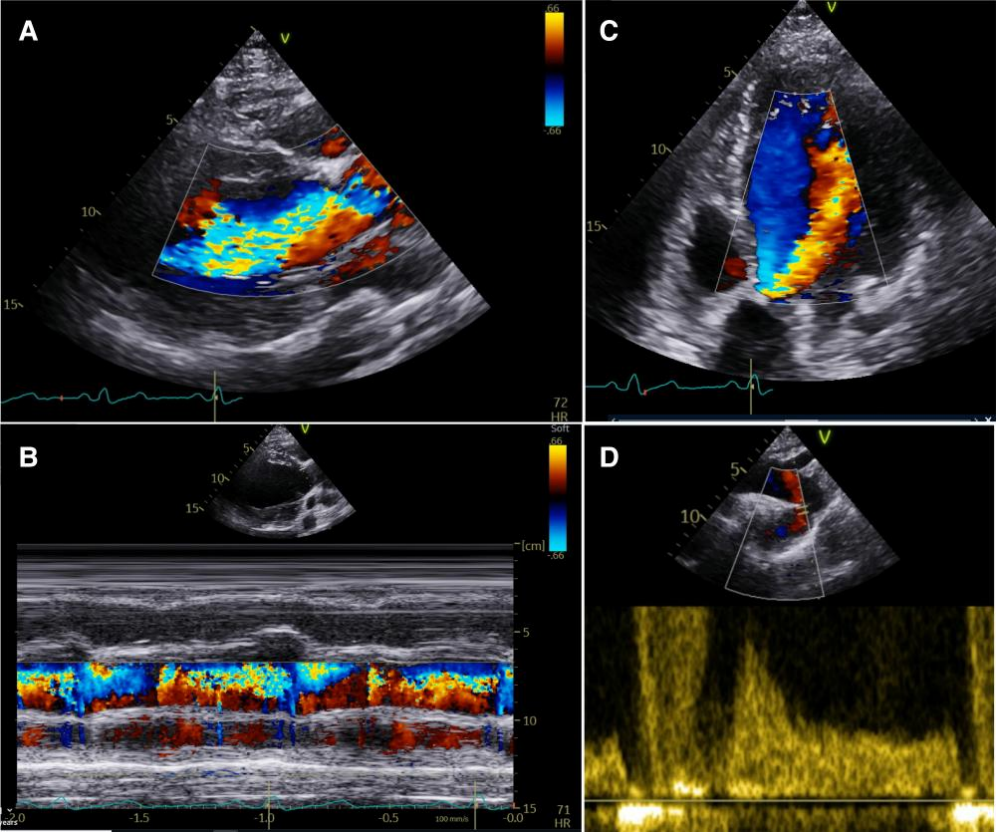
Echocardiography for AR quantification. (*A*) PLAX view with colour flow Doppler showing severe AR. The colour baseline can be shifted in order to measure the proximal isovelocity surface area. (*B*) M-mode with colour flow Doppler transecting the AR jet in the PLAX view to measure the proportion of the left ventricular outflow tract filled by the AR jet. (*C*) Apical 5 chamber view demonstrating jet of severe AR. (*D*) Pulsed wave Doppler measured in the proximal descending aorta showing holodiastolic flow reversal.
